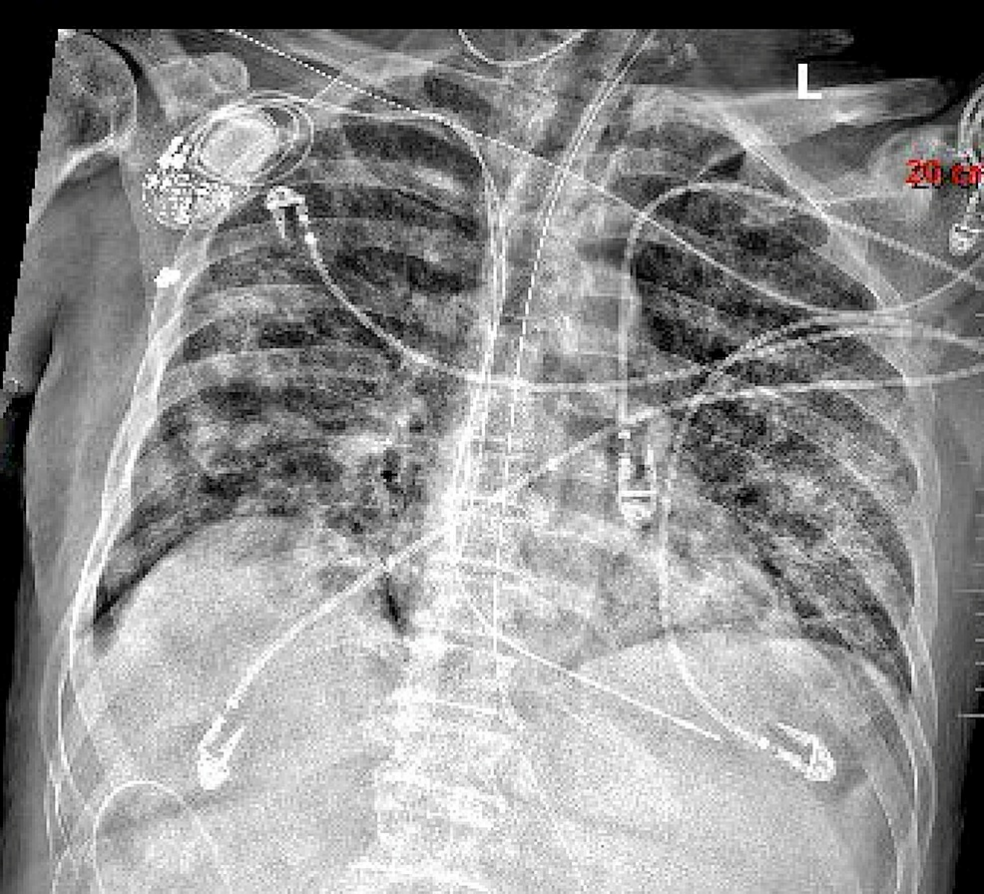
Chest x-ray on the day of patient’s death showing elevation of the right hemidiaphragm and worsening patchy bilateral airspace disease
